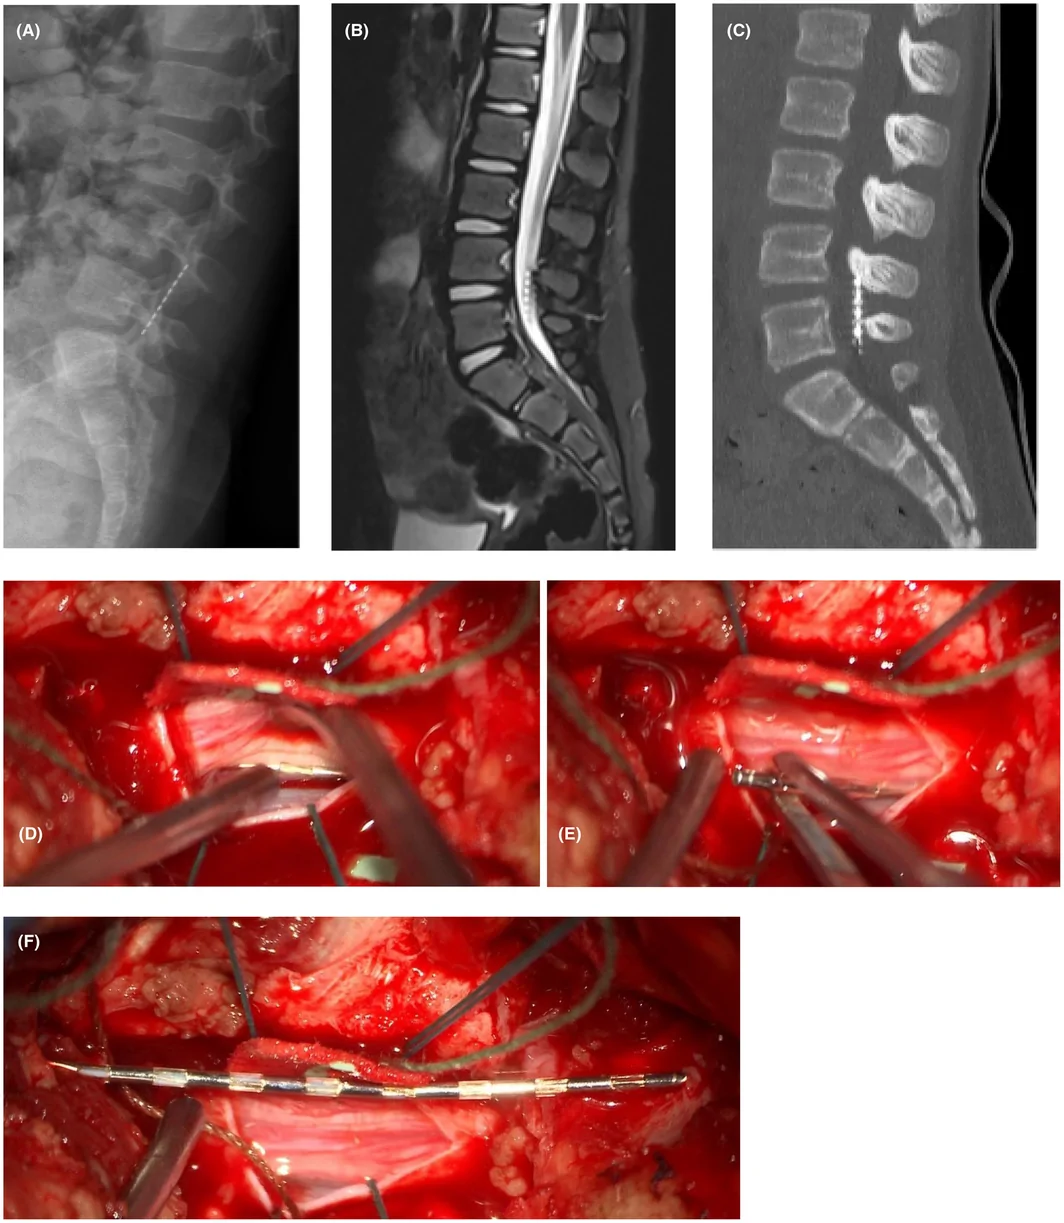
Spinal migration and surgical findings. (A) Sagittal X‐ray showing electrode position at the L4–L5 level. (B) Sagittal spine MRI showing retained SEEG electrode within the spinal canal at the L4–L5 level, in contact with the cauda equina. (C) Sagittal CT showing electrode position. (D–F) Intraoperative photograph showing the removal of the retained electrode in the spinal canal.
